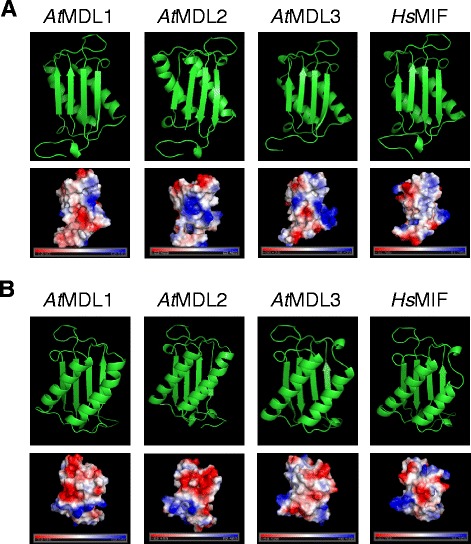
Predicted *At*MDL 3D structures. Amino acid sequences of the *At*MDL proteins were subjected to analysis *via* the PHYRE^2^ Fold Recognition server (http://www.sbg.bio.ic.ac.uk/phyre2/html/page.cgi?id=index) and rendered with PyMOL (http://www.pymol.org/). The predicted 3D structures (ribbon models and electrostatic surface potential) of *At*MDL1, *At*MDL2 and *At*MDL3 are visualized in comparison to the known X-ray-resolved 3D structure of *Hs*MIF. In the upper panels, green arrows symbolize β-sheets and green coils α-helices. In the lower panels, red color indicates an excess of negative and blue color an excess of positive charges near the surface, while white color specifies neutral regions. For simplicity, the structure of the monomer is shown only. A View with the four-stranded β-sheet in front. B View with the two antiparallel α-helices in front.
